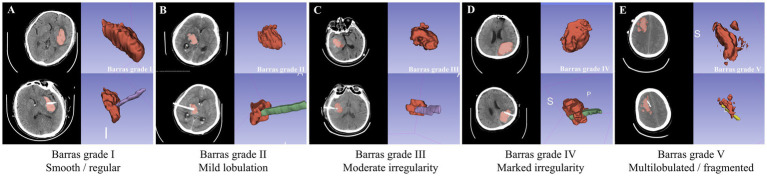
Representative Barras morphology grades on axial CT and 3D reconstructions. (A–E) show Barras grades I–V, respectively: grade I, smooth/regular; grade II, mild lobulation; grade III, moderate irregularity; grade IV, marked irregularity; and grade V, multilobulated or fragmented morphology. The left subpanels show axial non-contrast CT with segmented hematoma, and the right subpanels show corresponding reconstructions generated using 3D Slicer. Where visible, the catheter trajectory is indicated on postoperative images.
